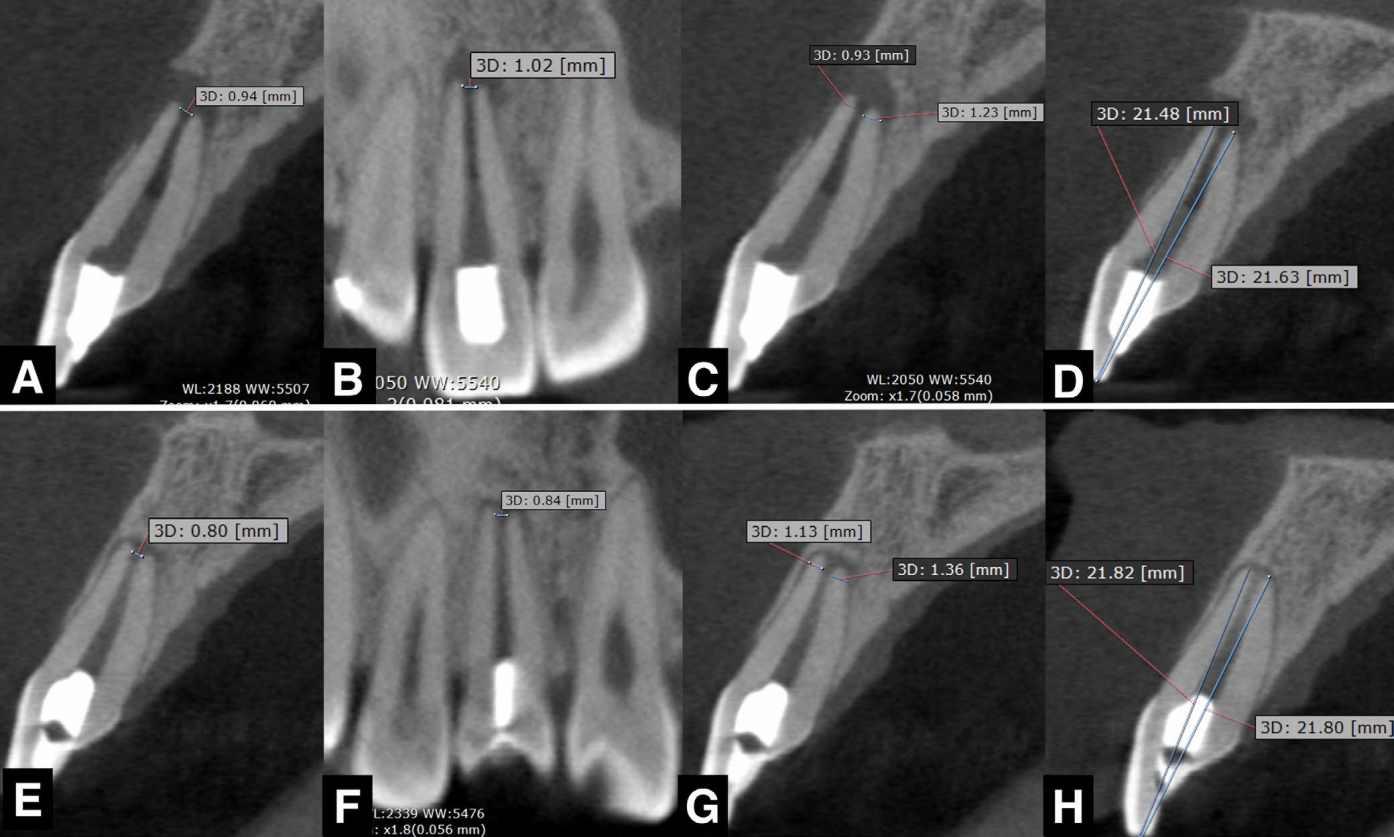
CBCT measurements of tooth #11. Pre‐treatment: (A) Foraminal diameter (sagittal view) = 0.94 mm; (B) Foraminal diameter (coronal view) = 1.02 mm; (C) Dentin thickness (buccal = 0.93 mm, palatal = 1.23 mm); (D) Root length = 21.48–21.63 mm. Post‐treatment: (E) Foraminal diameter (sagittal view) = 0.80 mm; (F) Foraminal diameter (coronal view) = 0.84 mm; (G) Dentin thickness (buccal = 1.13 mm, palatal = 1.36 mm); (H) Root length = 21.82–21.80 mm.
